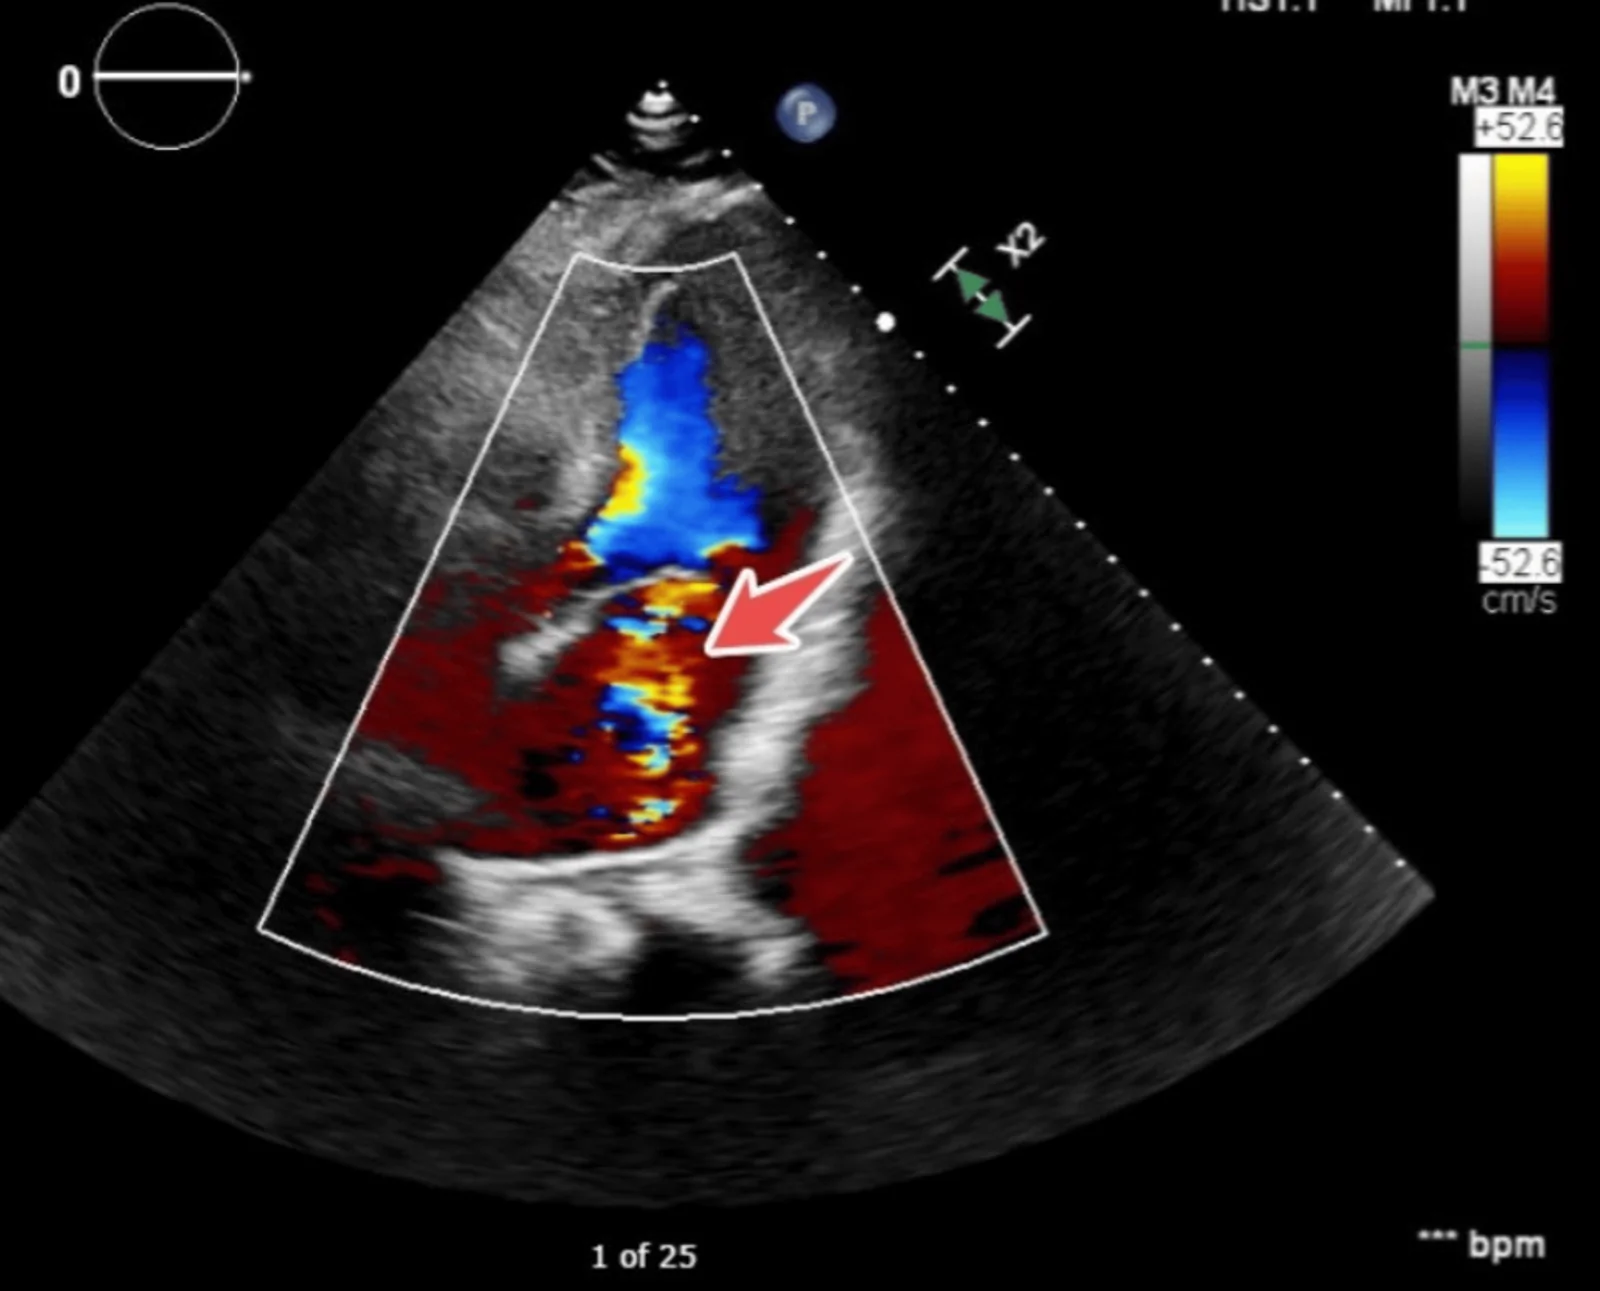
Severe eccentric anteriorly directed mitral regurgitation on color doppler echocardiography (arrow indicates the regurgitant jet)
